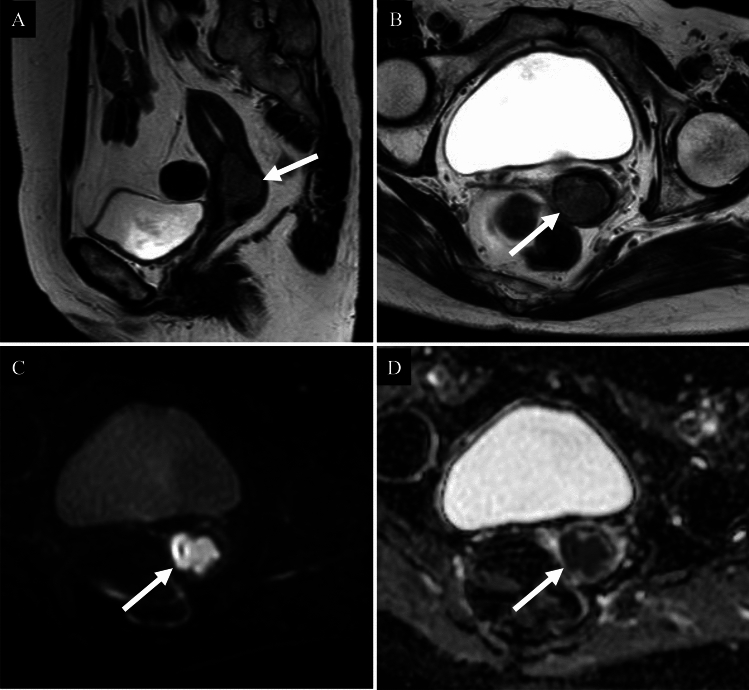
Usual type adenocarcinoma of the uterine cervix in a woman in her sixties. A Sagittal T2-weighted image; B Axial T2-weighted image; C Axial diffusion-weighted image; D Axial apparent diffusion coefficient map. A well-defined expansile cervical mass with high signal intensity relative to the surrounding stroma is observed on T2-weighted image (A, B: arrows). The lesion demonstrates a markedly high signal on diffusion-weighted image (C: arrow) and significantly reduced apparent diffusion coefficient values (D: arrow), with a mean ADC of 0.674 × 10⁻^3^ mm^2^/s. The posterior right cervical stroma appears thin but preserved, with no imaging evidence of parametrial infiltration. Pathological examination confirmed FIGO 2018 stage IB1 usual type HPV-associated adenocarcinoma
